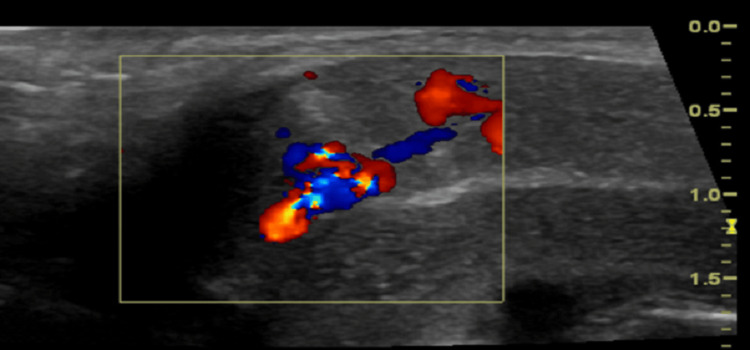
Doppler ultrasound image from case 2 showing turbulent, high-velocity arterial flow consistent with an arteriocavernosal fistula in the right corpora cavernosa
